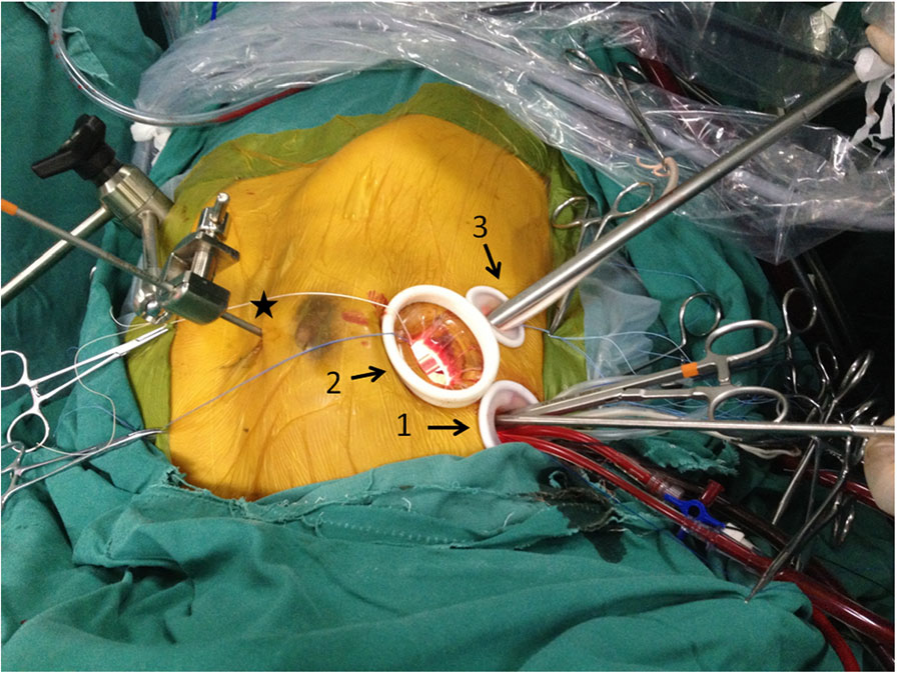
The operative layout for the totally thoracoscopic mitral valve procedure. Drainage of the superior vena cava and right superior pulmonary vein, and cardioplegia perfusion cannula was passed through port 1 directly. Port 2 was located in the fourth intercostal space on a midclavicular line for the entry of instruments, such as scissors and prosthesis, or tissue removal. Port 3 was located in the fifth intercostal space on the right anterior axillary line (arrows). The left atrium was raised by a blade retractor and introduced through a 2-mm shaft penetrating parasternally in the fourth intercostal space and was held on the operating table (asterisk)
